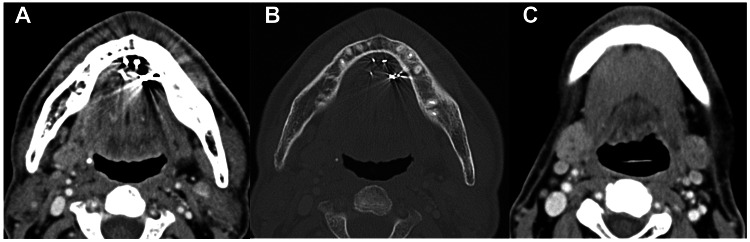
Contrast-enhanced CT four months after the implant. Detection of gold grains in the left floor of the mouth in soft tissue (A) and bone (B) window images. Soft tissue window image at the submandibular gland level (C). CT: Computed tomography
